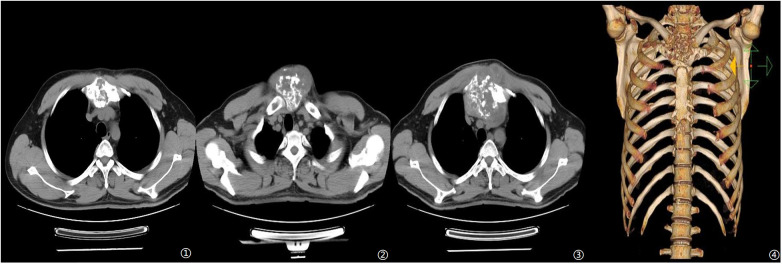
CT before operation. ① Initial CT scan in 2023. ②③④ Preoperative CT scan and Three-dimensional reconstruction CT.
